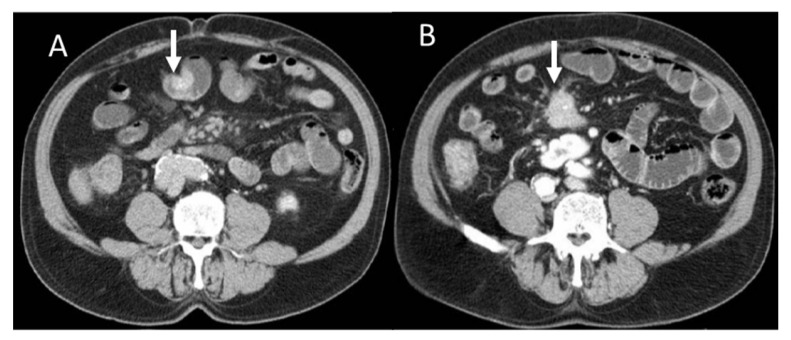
Portal CT evaluation of small bowel NEN (arrow in (A)): intraluminal enhanced polypoid lesion (arrow) with desmoplastic reaction (arrow in (B)).
